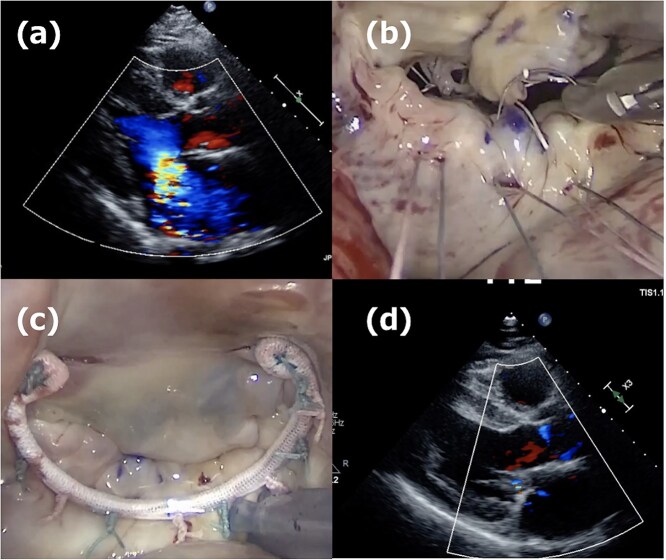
First operation images. (a) Severe mitral regurgitation caused by A2 prolapse. (b) Intraoperative image showing the placement of four 22-mm ePTFE loops fixed to the A2 segment with 5/0 polypropylene sutures. (c) The 36-mm CG-future band (Medtronics) was applied for mitral annuloplasty. (d) One week postoperative echocardiogram showing no residual mitral regurgitation.
